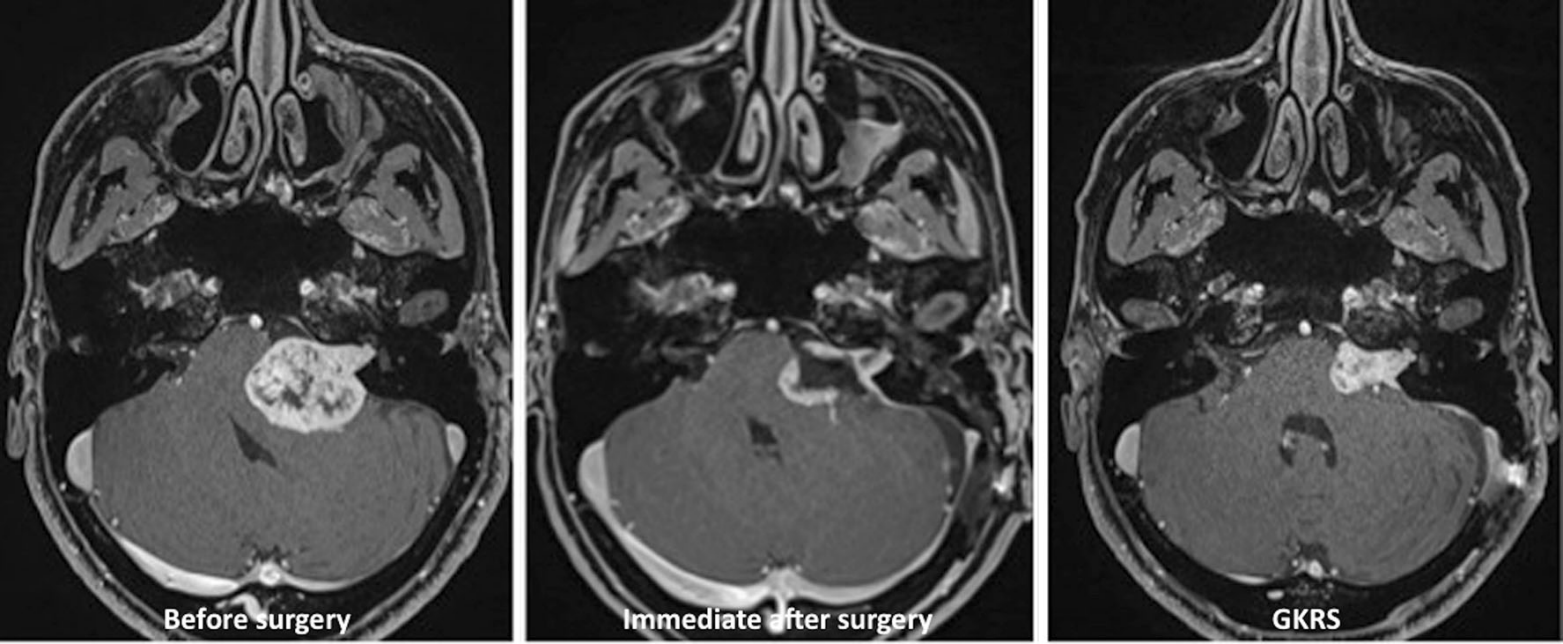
Representative axial images of T1-weighted contrast-enhanced MRI showing the preoperative size of the tumor (*left*), the residual capsule immediately after surgery (*center*), and the folding of the capsule and reduction of mass effect on the brainstem at the time of GKRS (*right*), which results in a compact and small target volume
